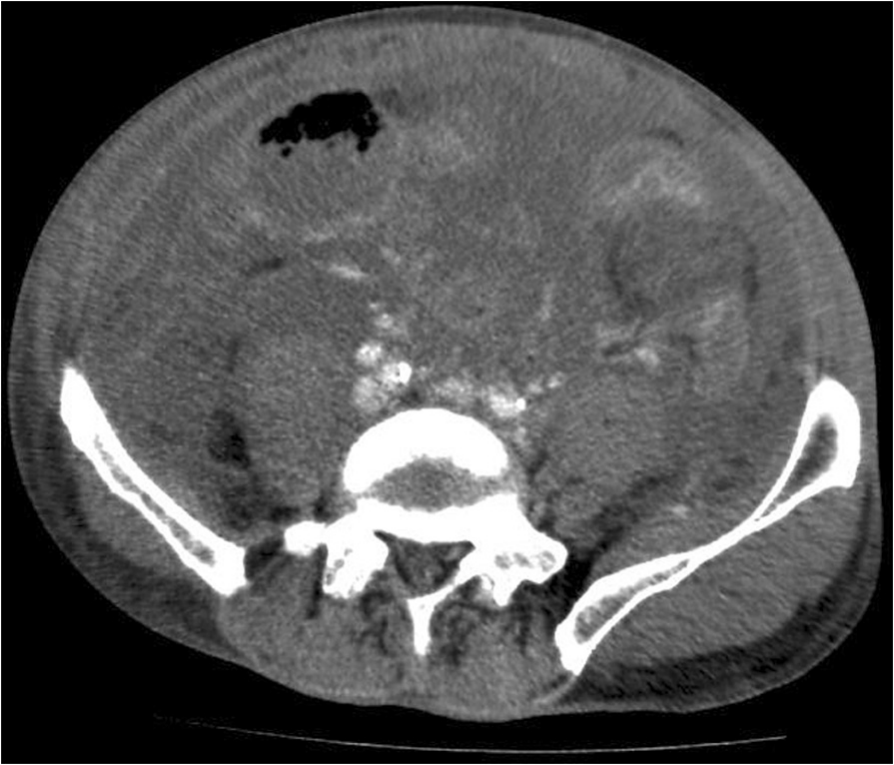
CT abdomen (patient 1), pelvic level, where the severely oedematous peritoneum and mesentery are filling the abdominal cavity.
